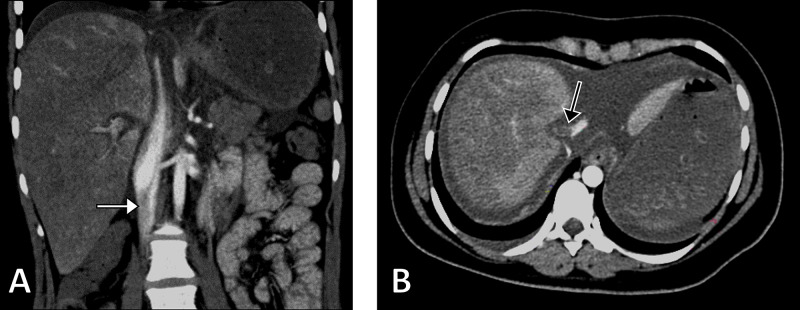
Computed Tomography Images Coronal (A) and axial (B) computed tomography images demonstrating a filling defect in the inferior vena cava (white arrow) and the hepatic vein (black arrow).
